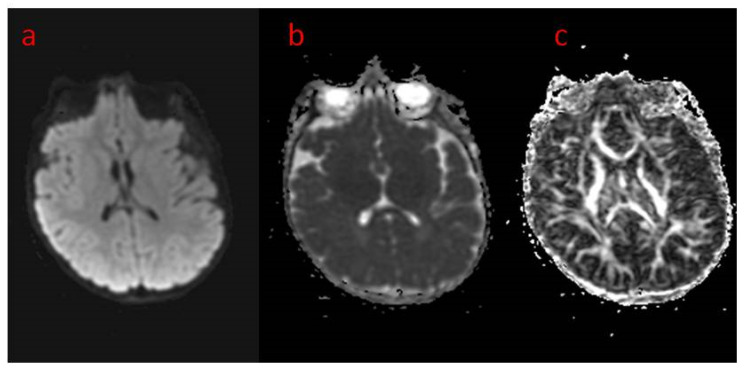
(a–c) Brain MRI in advanced sequences: (a) DWI diffusion weighted imaging; (b) ADC map—apparent diffusion coefficient map—areas of prolonged diffusion in peritrigonal white matter regions corresponding to unfinished myelinisation, (c) FA—fractional anisotropy sequence—symmetrical image of fibers of both hemispheres.
